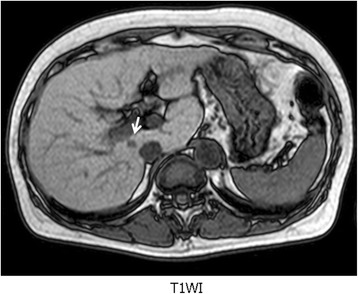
A small hepatocellular carcinoma (HCC) (diameter 5 mm) (*white arrow*) was detected in the paracaval caudate lobe of a 63-year-old female patient during a regular follow-up magnetic resonance imaging examination in 2012
